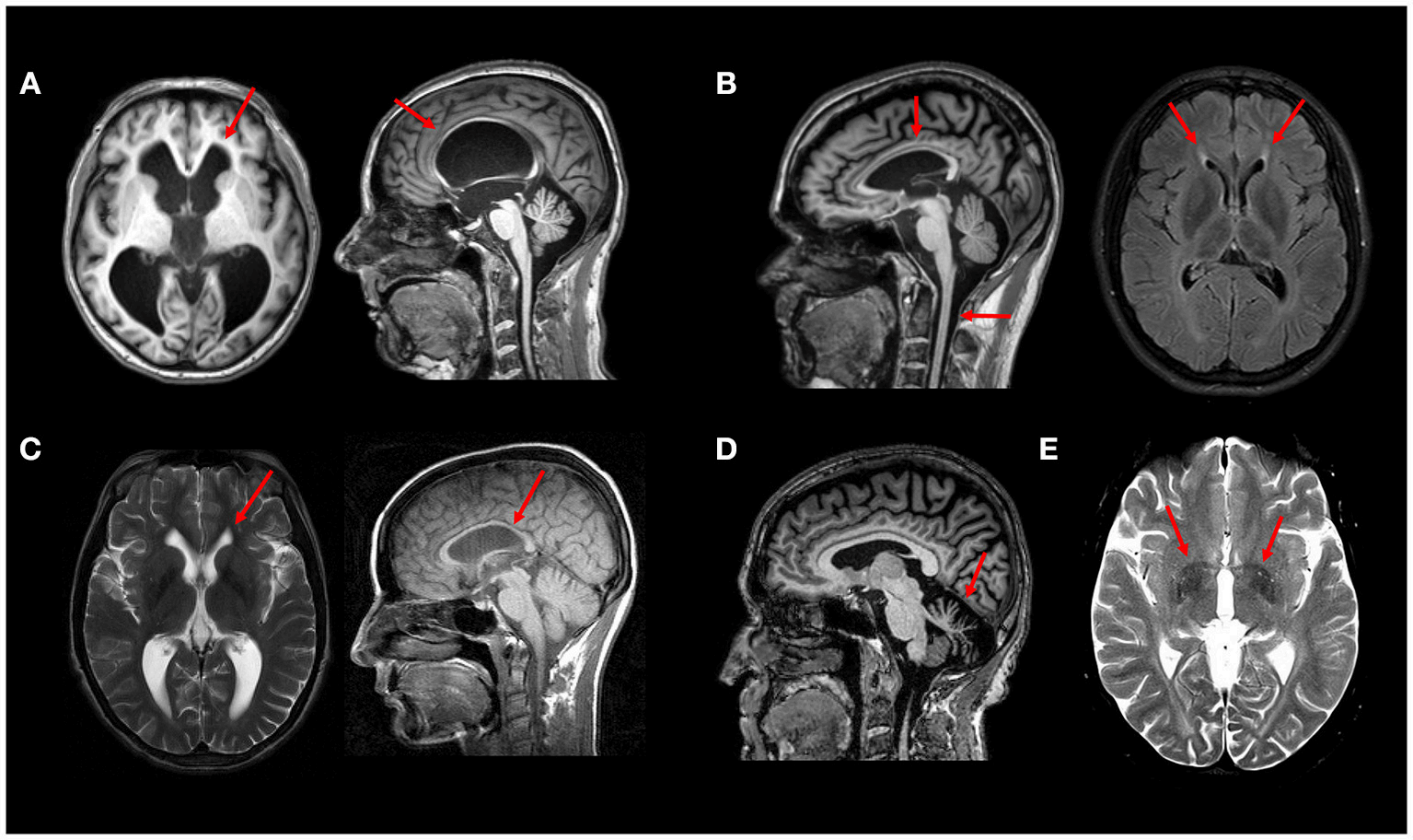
Typical MRI findings in distinct HSP subtypes—(A) Axial and sagittal T1W images showing hydrocephalus due to aqueductal stenosis in a patient with SPG4. This finding is rather unusual in SPG4, but relatively frequent in SPG1 patients. (B) Sagittal T1W image showing thin corpus callosum and spinal cord atrophy in a patient with SPG11; Axial FLAIR image showing the “ear-of-the-lynx” sign in the same patient. (C) Thin corpus callosum (T1W image) and subtle “ear-of-the-lynx” sign (T2W image) in a patient with SPG15. (D) Sagittal T1W image revealing marked cerebellar atrophy in a patient with SPG7. (E) White matter hyperintensities and T2 hypointense signal at the globi pallidi in a 21-years old patient with SPG35 (T2W image). All images were obtained during routine clinical care of these patients at UNICAMP and UNIFESP hospitals.
